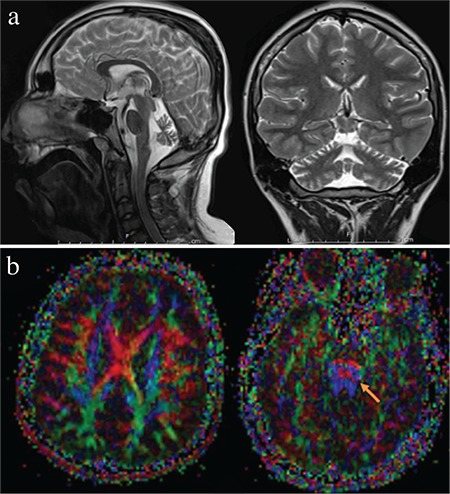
Sagittal and coronal brain magnetic resonance images of a 14-year-old boy revealed cerebellar vermian volume loss with normal pons and spinal canal (a). Diffusion tensor imaging images displayed thinning of the tegmental extending through corticospinal tracts (b).
